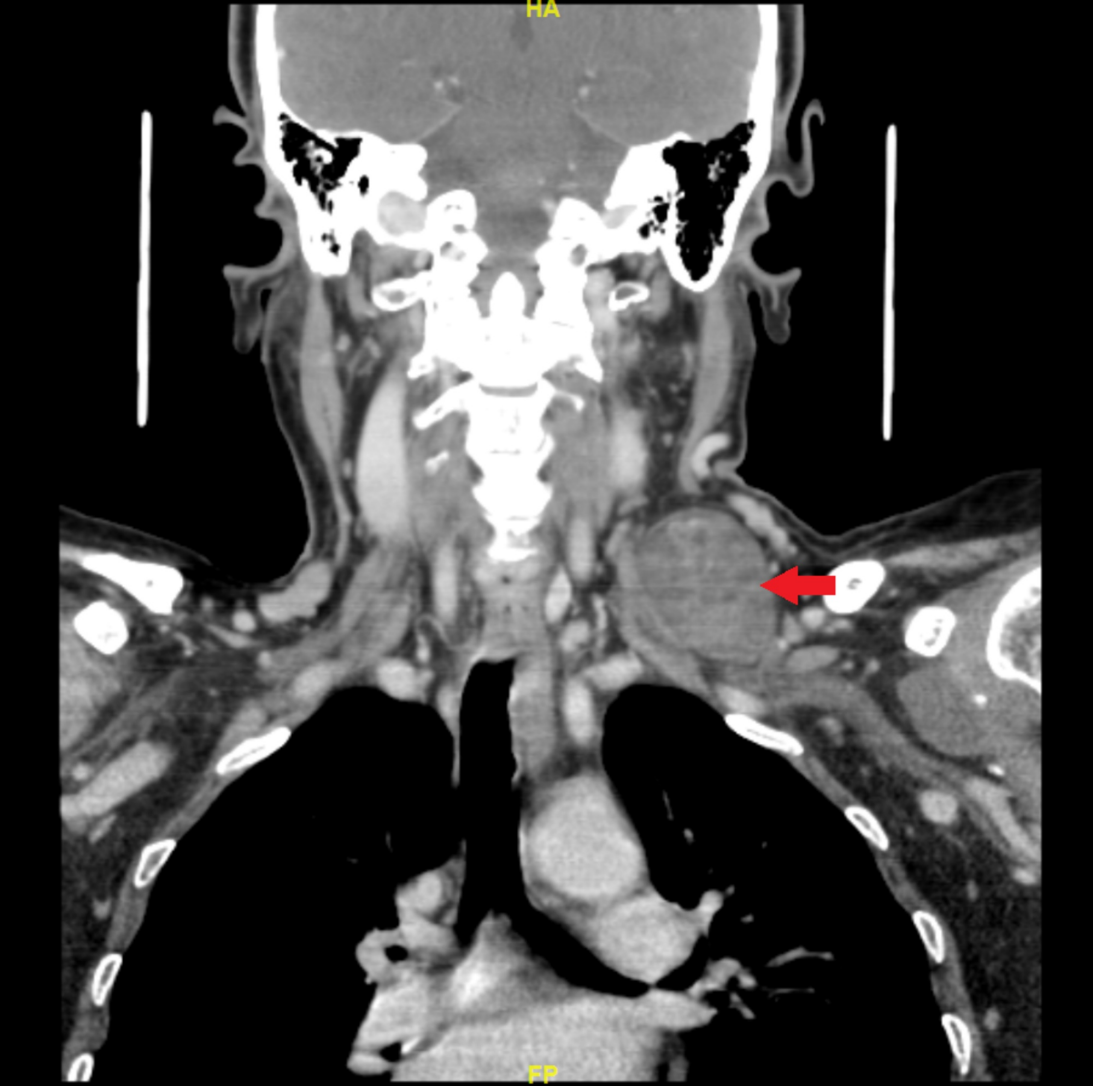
Contrasted coronal CT of the neck A left-sided supraclavicular well-circumscribed mass measuring 3.5 × 4 cm (arrow) CT, computed tomography
